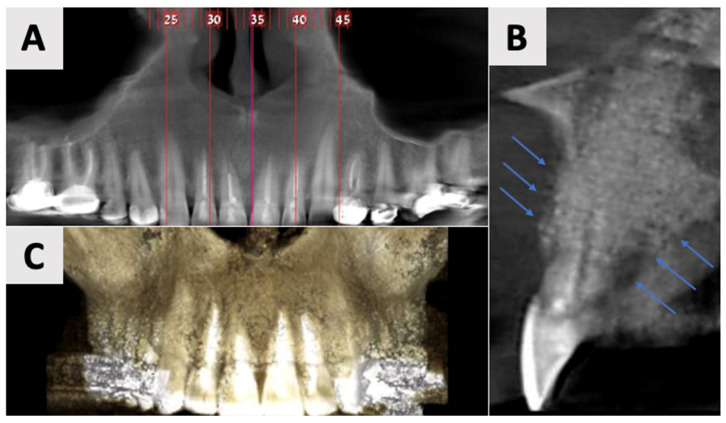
CBCT imaging analysis 2 months post-augmentation. (A) Panoramic reconstruction showing the overall healing of the defect site. (B) The restored contour of the facial and palatal bony walls (blue filled lines). (C) Paraxial view showing the initial osseous regeneration.
